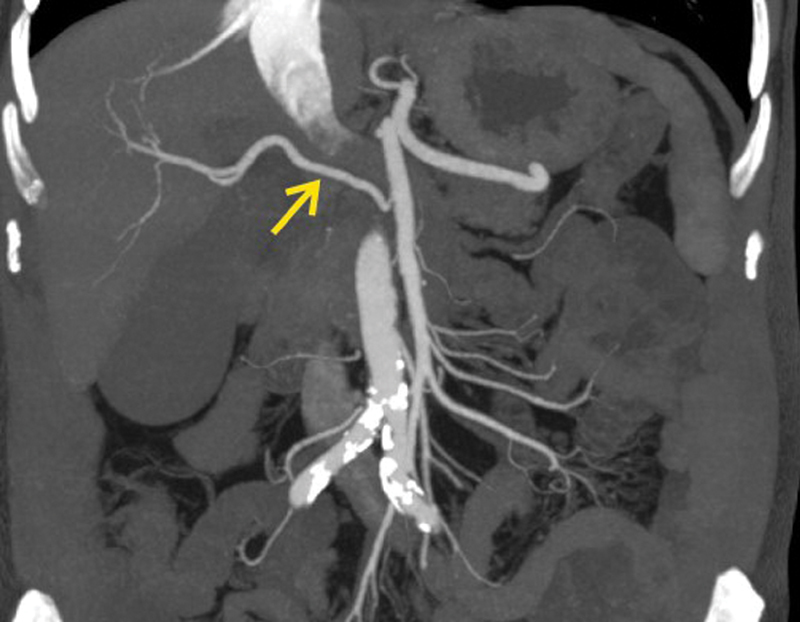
Coronal contrast-enhanced computed tomography scan arterial phase image showing replaced right hepatic artery (yellow arrow) arising from superior mesenteric artery.
